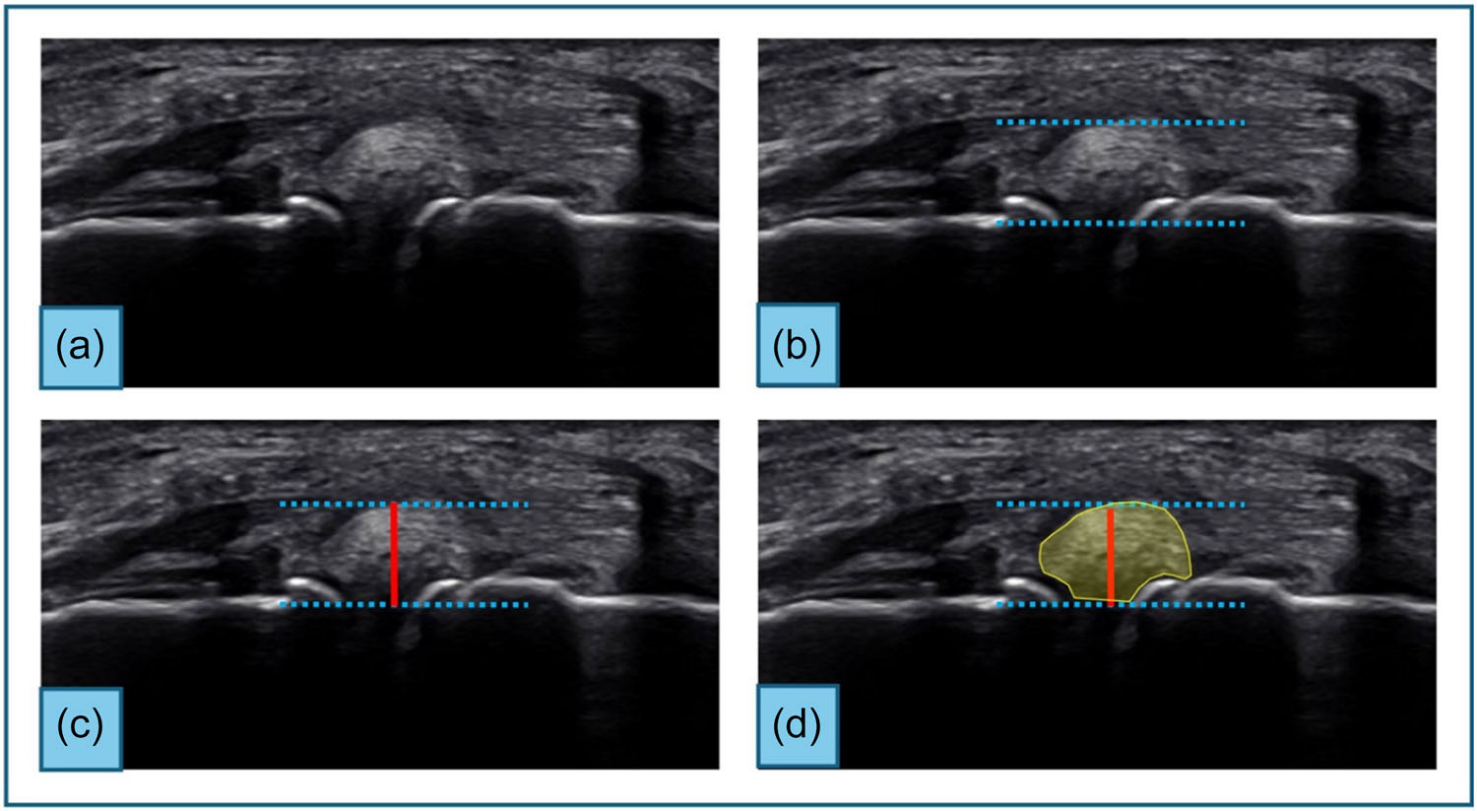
Measurement method of meniscus extrusion cross‐sectional area and distance. (a) Ultrasound image of an extruded meniscus without measurement outline. (b) Dotted blue lines are drawn tangent along femoral and tibial condyles & tangent drawn to the outermost edge of the meniscus. (c) The red line is drawn perpendicular to the dotted blue lines and represents the extrusion distance. (d) The yellow shade represents the extrusion cross‐sectional area.
